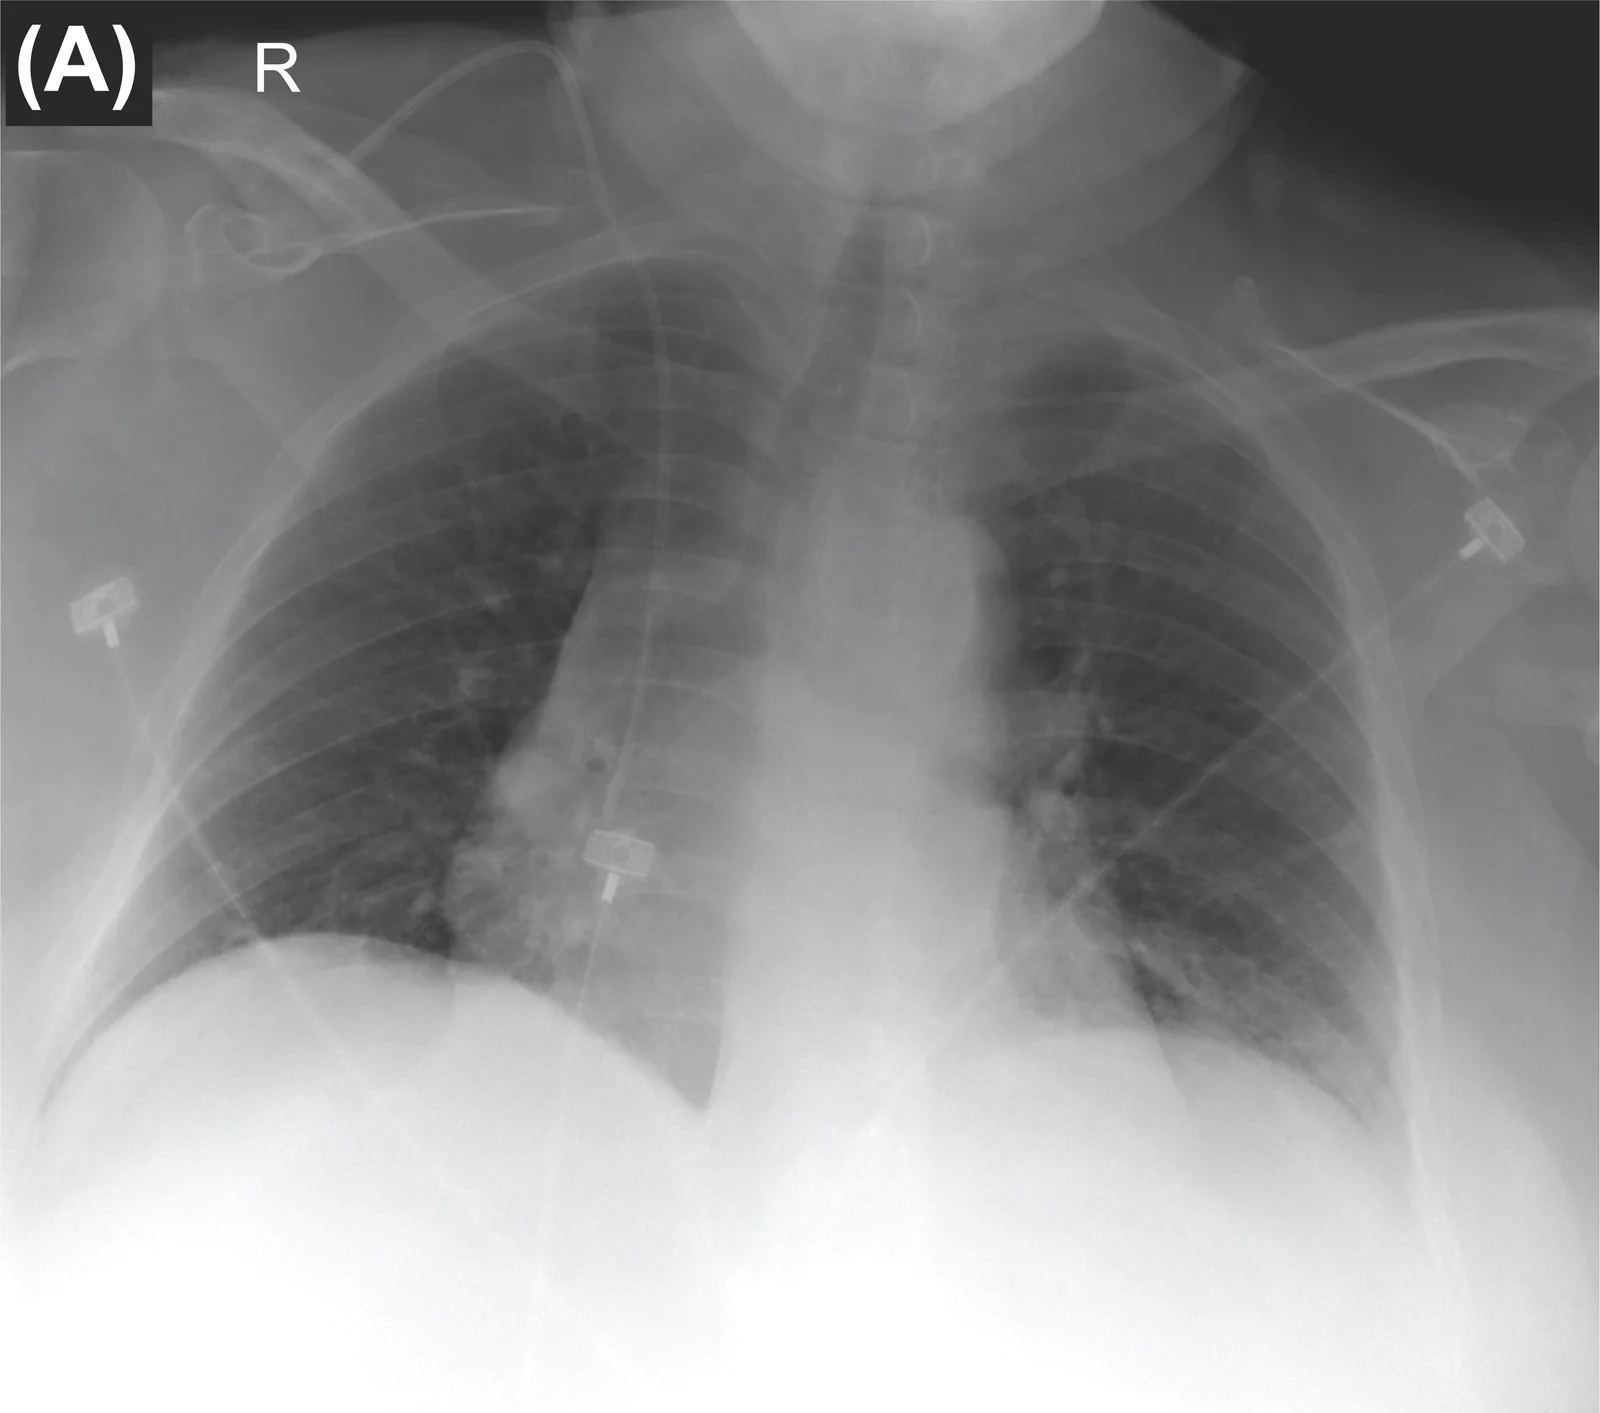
Chest radiograph. Bilateral hypoventilation with left basal reticular opacities and a small left pleural effusion; an indwelling central venous catheter *in situ*.
